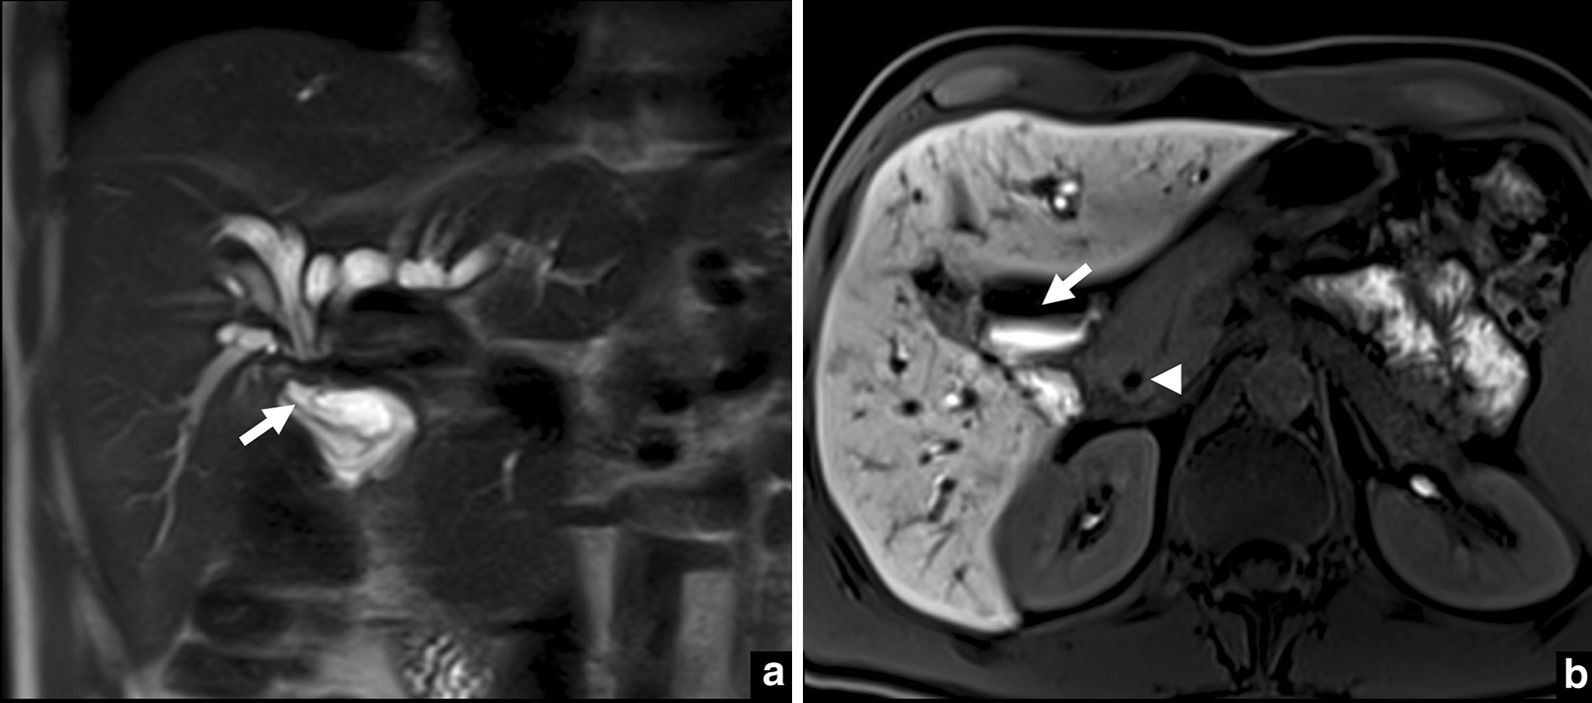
MRI one year after cholecystectomy. Coronal HASTE (a) shows intra-hepatic bile duct dilation with an abrupt stop at the confluence, in close contact with the duodenum (arrow). In the hepatobiliary phase (b) contrast was excreted to the duodenal bulb (arrow) and no contrast was depicted in the choledochus (arrowhead), confirming fistulisation
